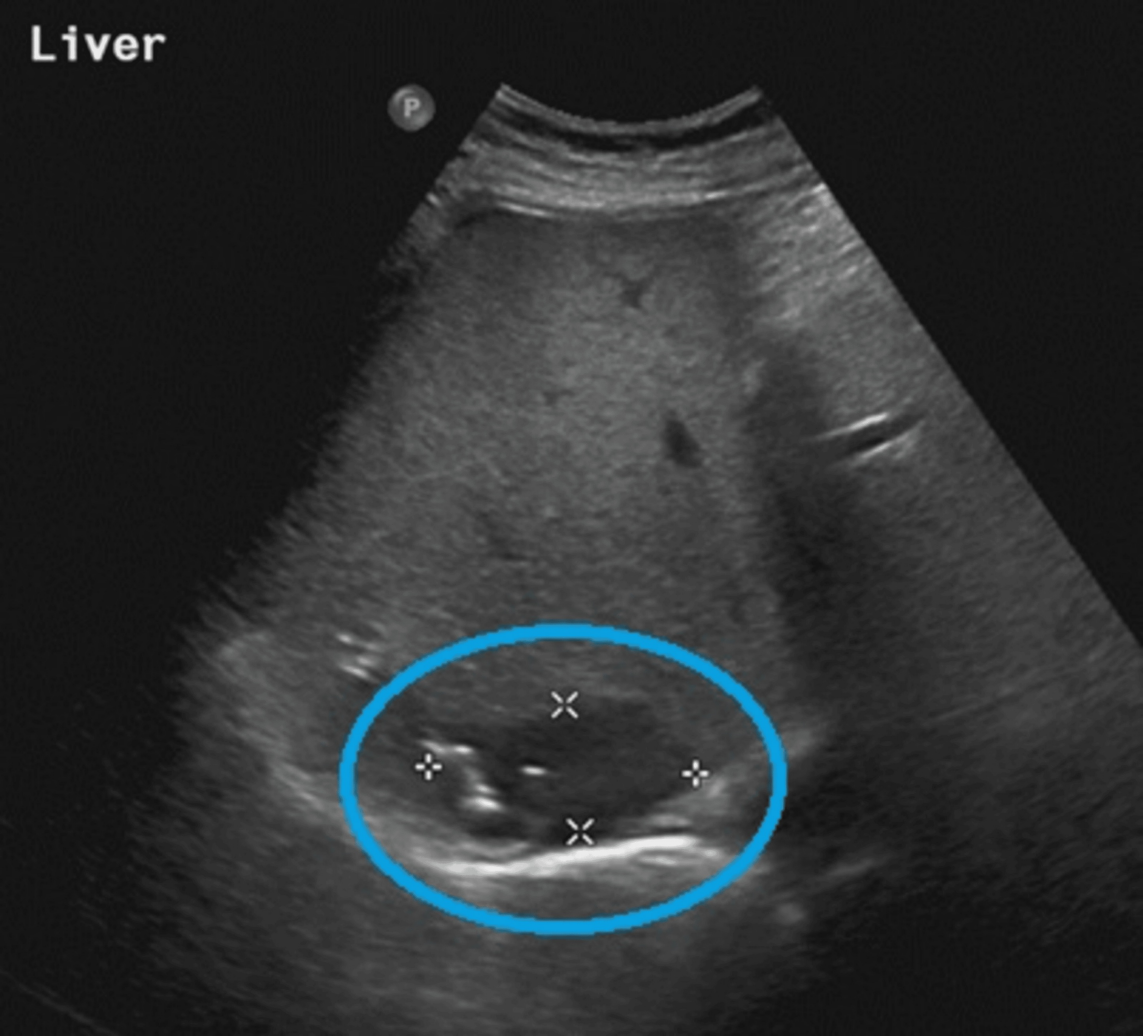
Ultrasound liver shows marked reduction of size of abscess (indicated by circle).
